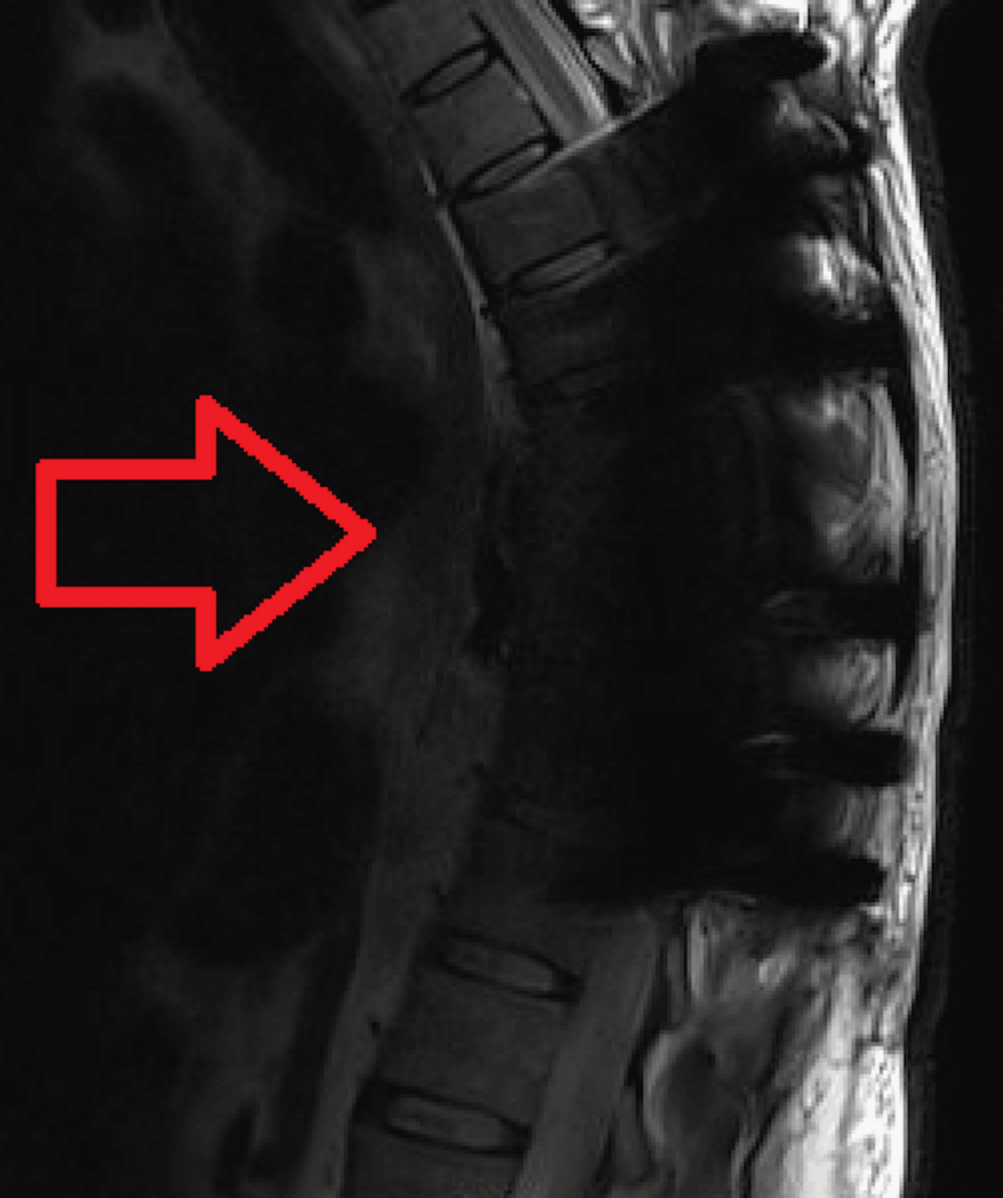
MRI acquired before discontinuation of IV antibiotics.
